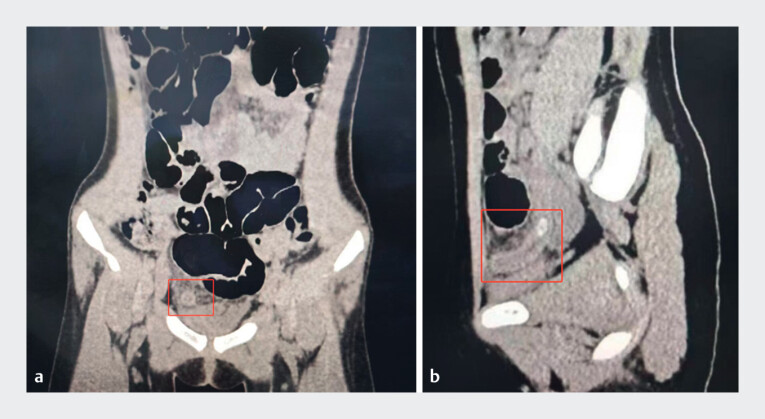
Computed tomography scan images showing:
